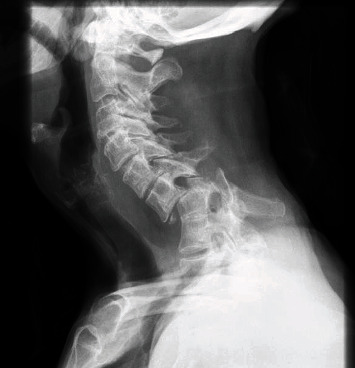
Preoperative X-rays (lateral view).
